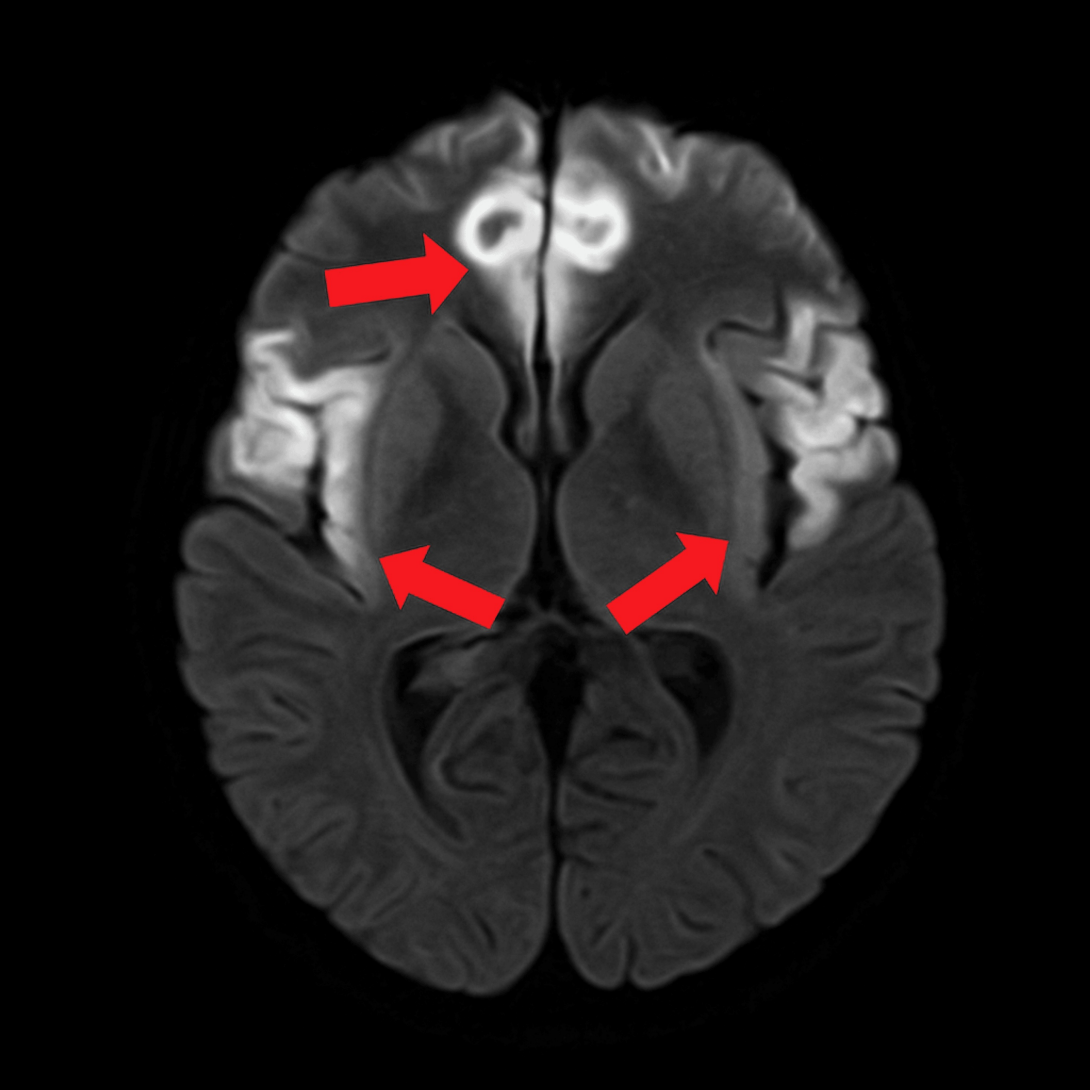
Axial DWI MRI of the brain Cerebral cortical restricted diffusion, as denoted by the red arrows, is indicated by the hyperintense and curvilinear regions most prominent in close proximity to the paramedian frontal lobes and subcortical regions, consistent with toxic-metabolic or anoxic injury. DWI: diffusion-weighted imaging, MRI: magnetic resonance imaging
